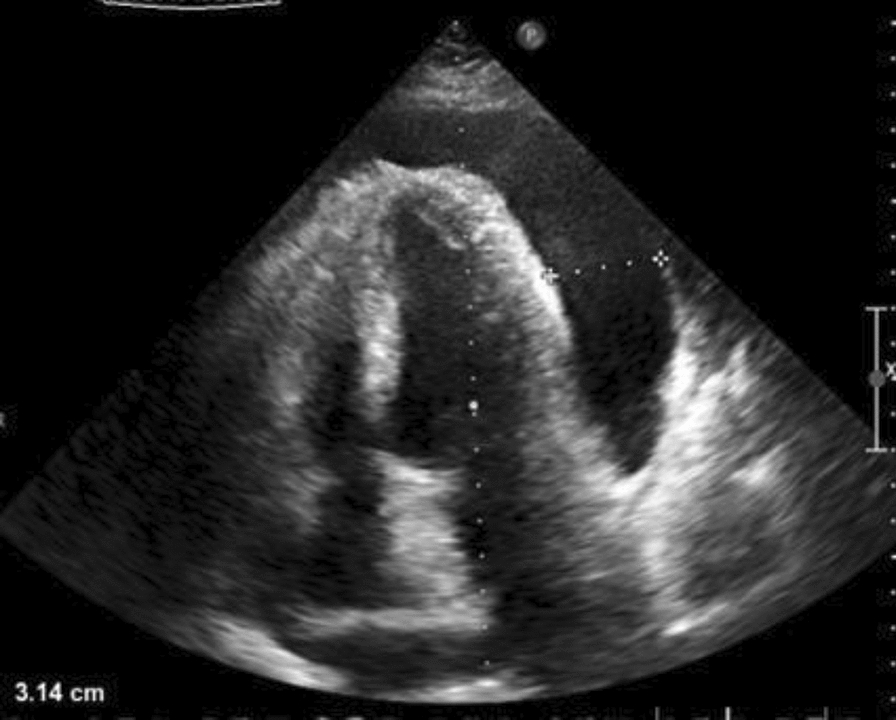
Transthoracic echocardiogram showed severe pericardial effusion (tamponade)
